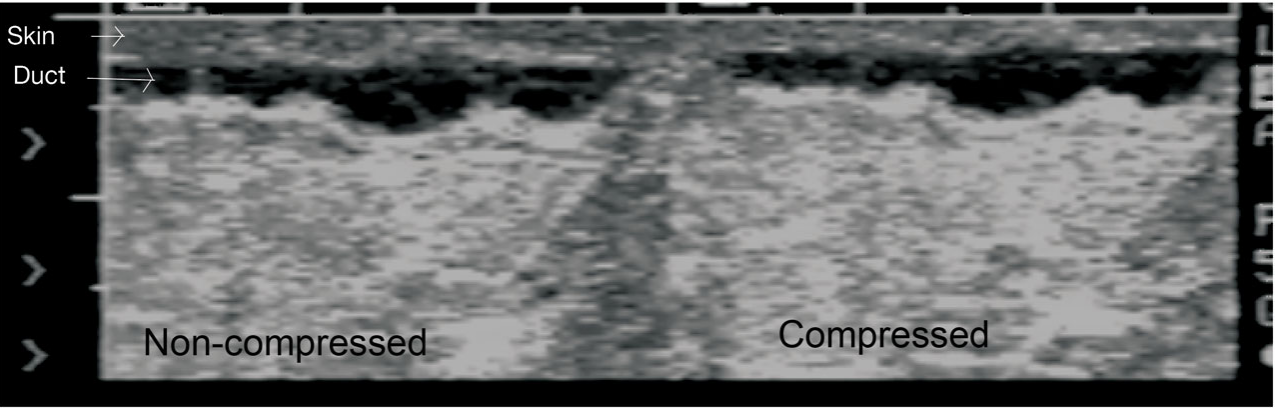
This blocked duct presented as a discrete mobile palpable lump. Ultrasonically it appears as a small hypoechoic tubular structure duct that is incompressible.
